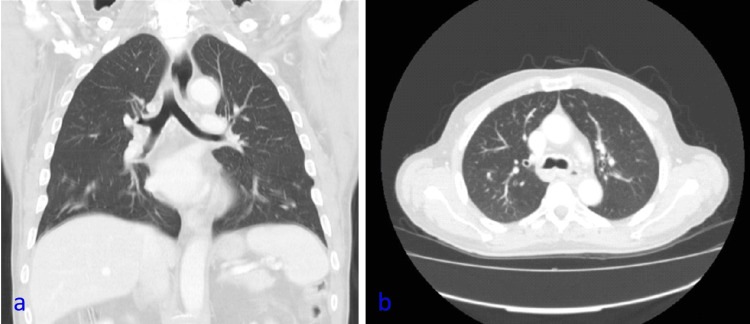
Chest CT scan a - coronal plane; b - axial plane: showing thickening of the esophageal wall and heterogeneity at the level of the carina
